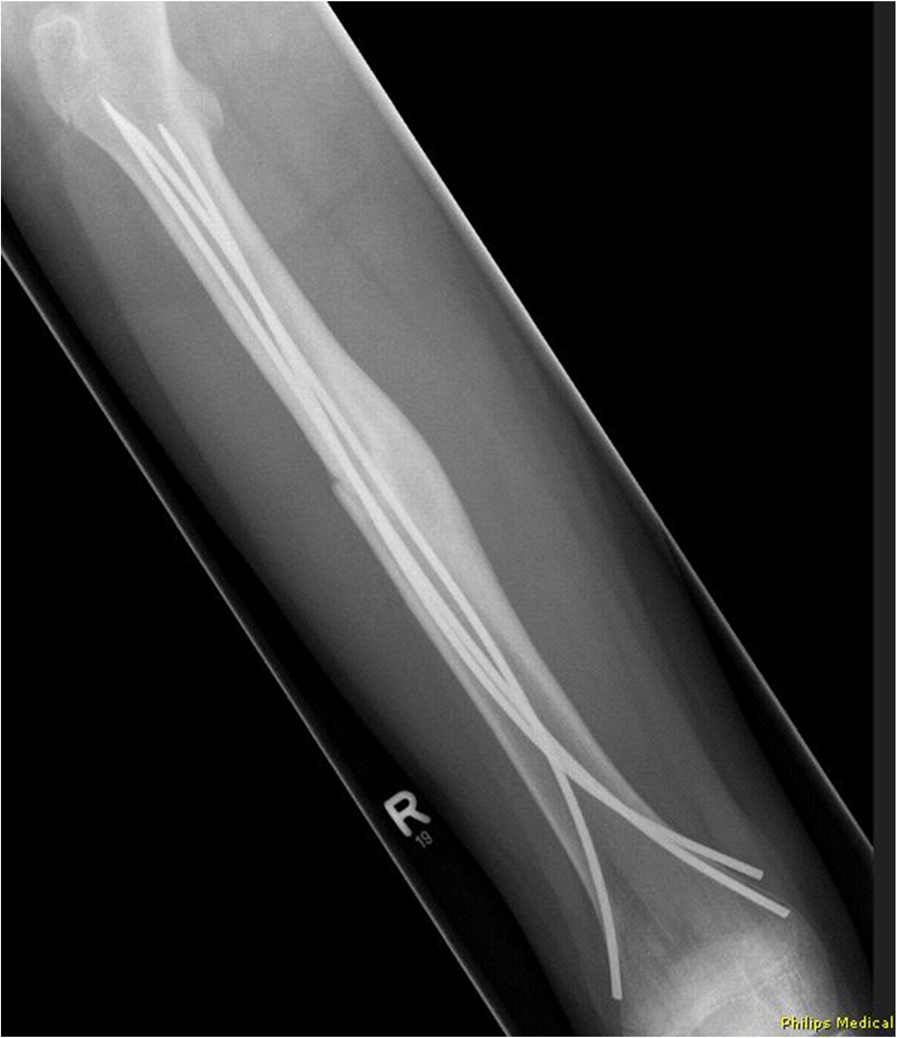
Radiograph (anterior-posterior view) of a 3CM configuration, 4 months postoperativly, diameter of the nails: 3 × 3.5 mm.
